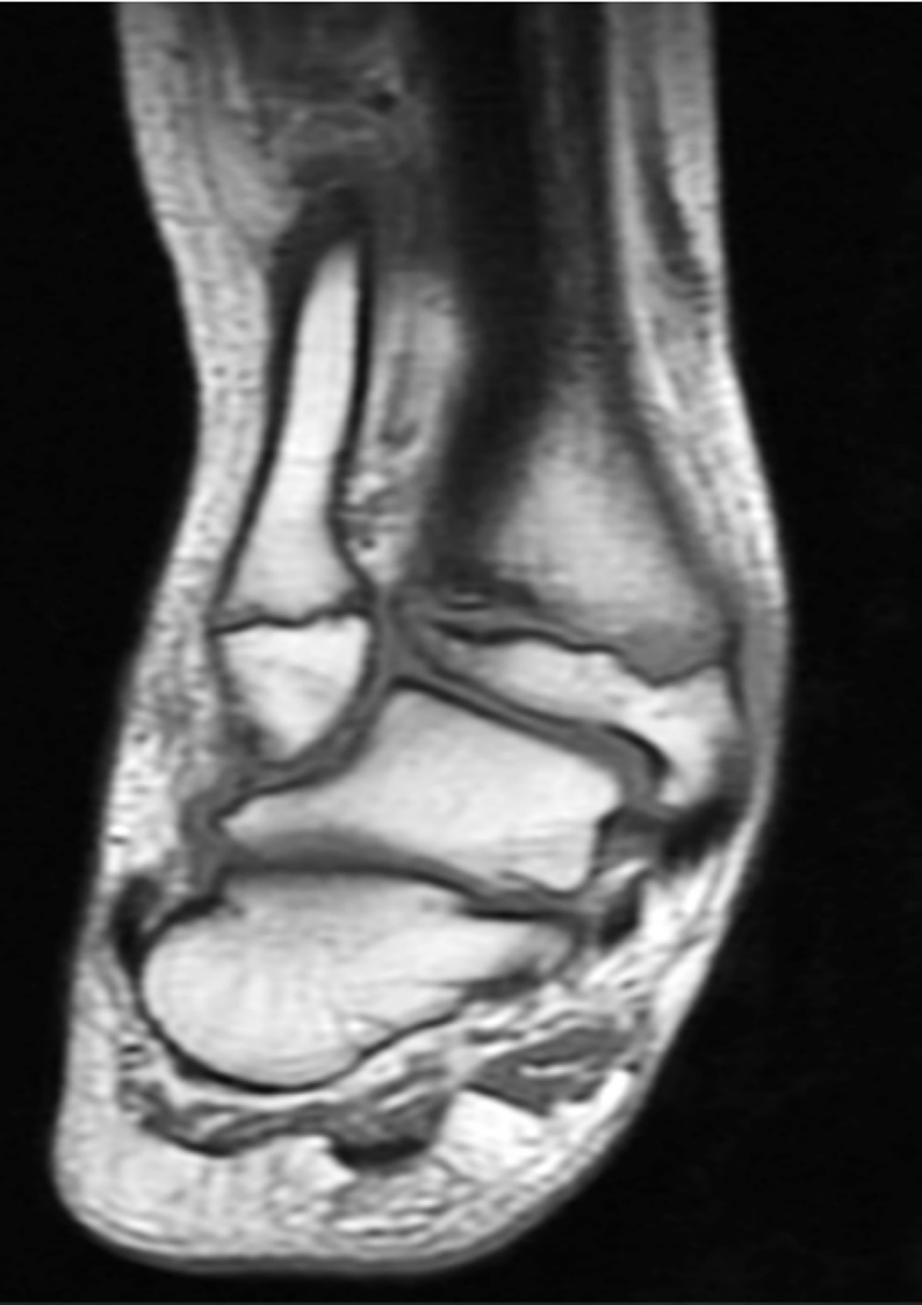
MRI of right ankle joint (coronal section) showing ankle valgus deformity.
